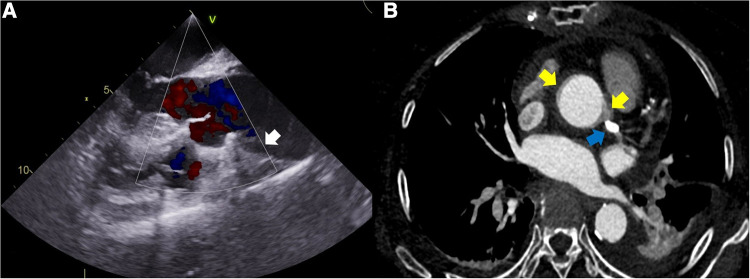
(A) Perioperative transesophageal echocardiography showing the flap of the proximal aortic dissection (white arrow). (B) Postoperative computed tomography showing a small remnant intramural hematoma (two yellow arrows) and the stent in the LAD (blue arrow). LAD, left anterior descending artery.
